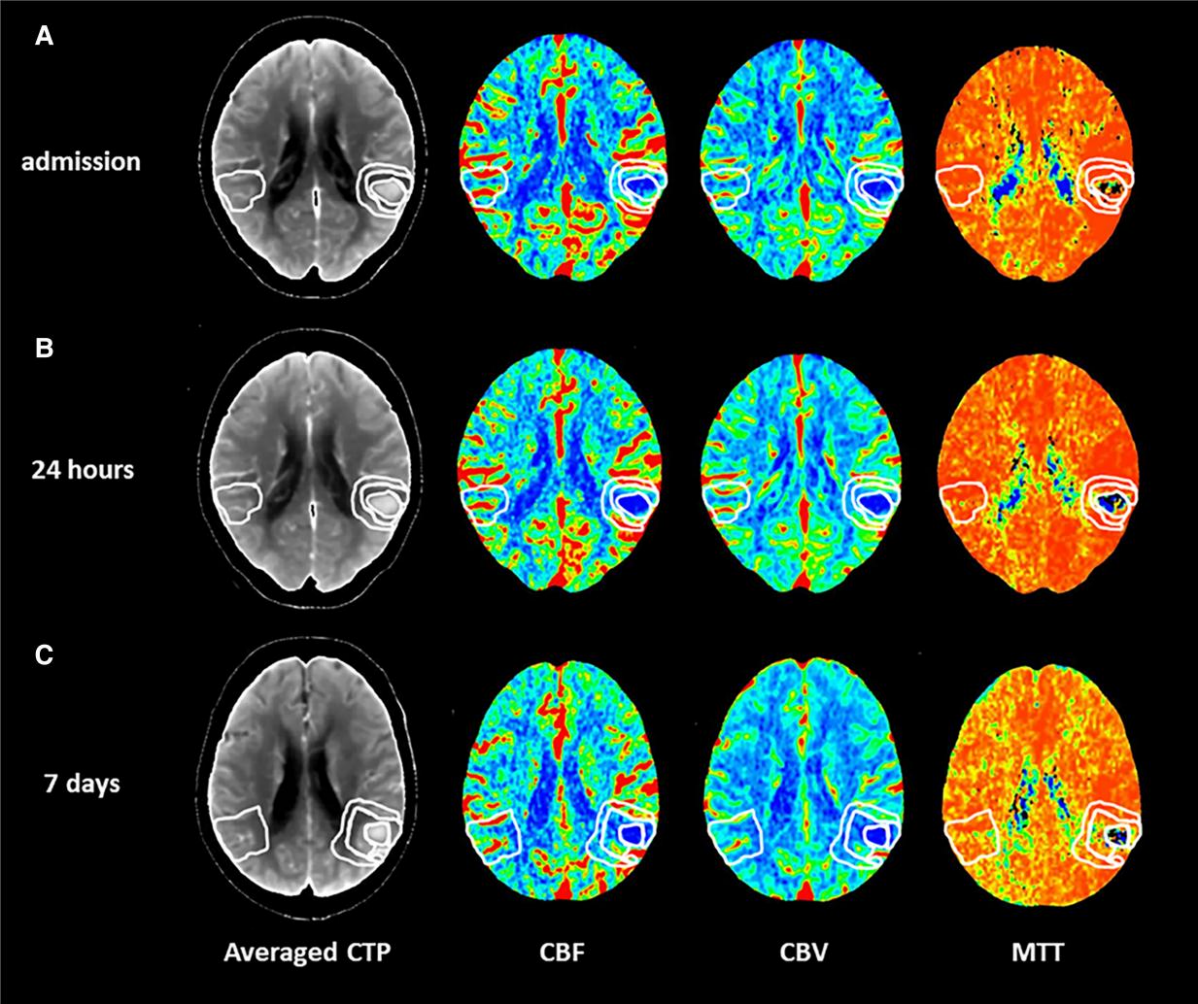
Perfusion mapping of acute spontaneous intracerebral haemorrhage. Computed tomography perfusion in a patient with acute spontaneous intracerebral haemorrhage located in the left temporal and parietal lobes performed at admission (A), at 24 h (B) and at 7 days (C) on averaged computed tomography perfusion images (averaged CTP). CBF, cerebral blood flow; CBV, cerebral blood volume; MTT, mean transit time.
